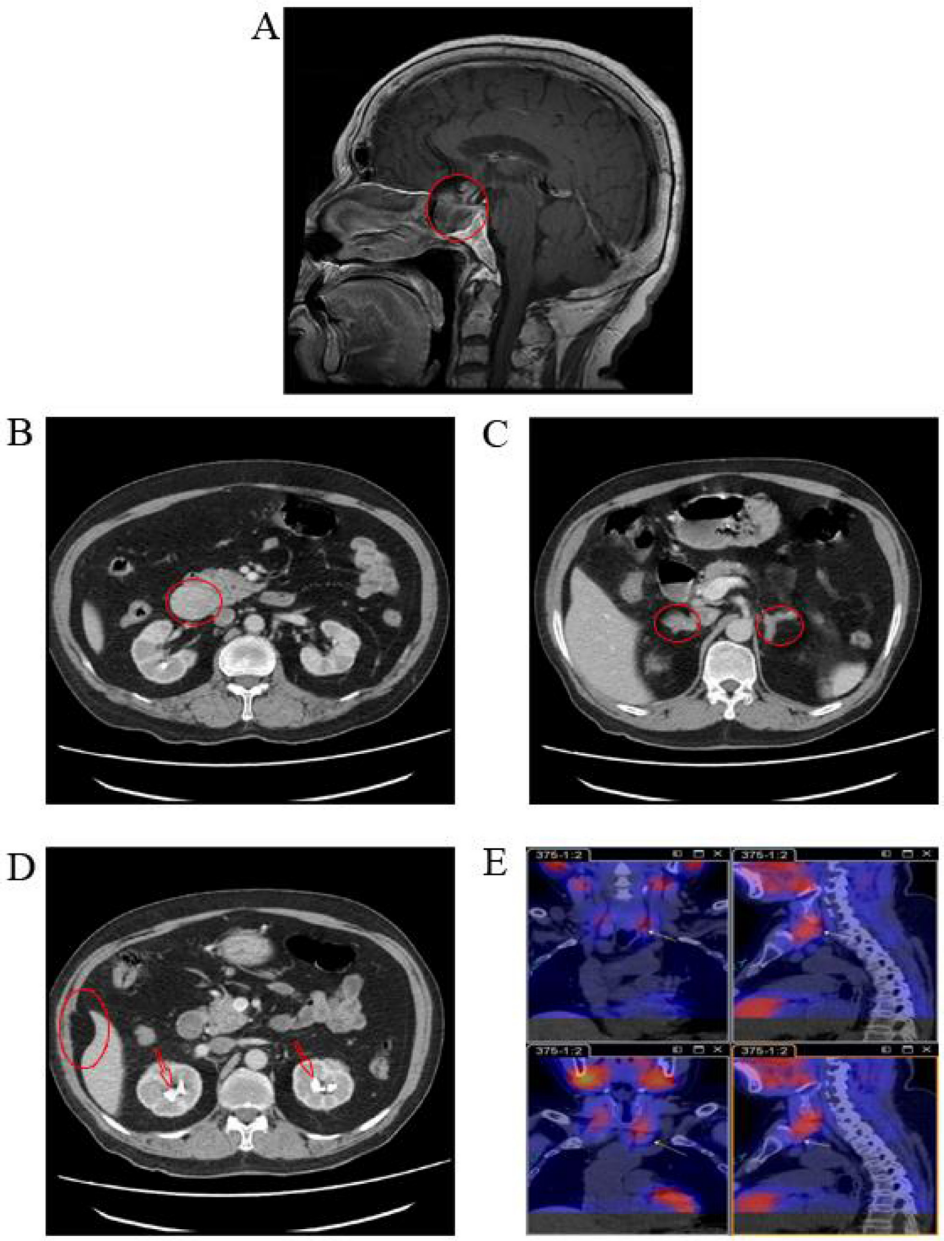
Medical imaging of the proband, II-3, in the *MEN1* syndrome family (A) MRI scans revealed a 2.0 × 2.5 × 2.5 cm pituitary tumor in the proband; (B, C, and D) Abdominal CT revealed a solid mass in the pancreatic head, bilateral adrenal nodules, bilateral renal stones, and abdominal lipoma in the proband; (E) SPECT revealed multiple nodules behind thyroid in the proband. MRI, magnetic resonance imaging; CT, computed tomography; SPECT, single photon emission computed tomography.
